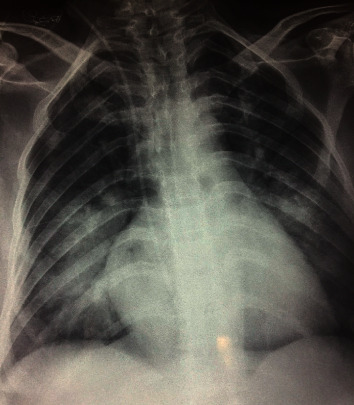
Admission Chest X-ray showing bilateral infiltrates.
